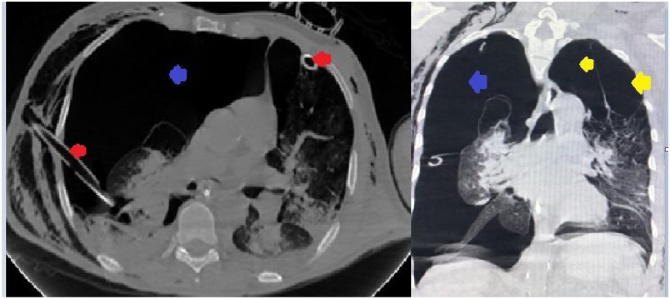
Thorax CT without contrast demonstrates subcutaneous emphysema, right lobe lung collapse, and absence of pneumothorax on the left side. Red arrows indicate chest tubes. Blue arrows indicate pneumothorax. Yellow arrows indicate bullae.
